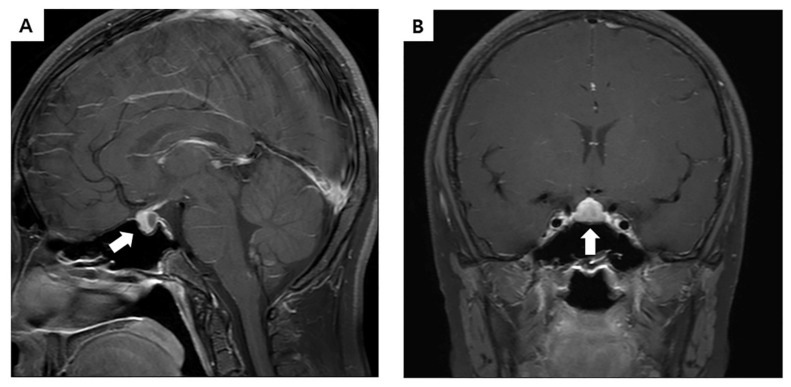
Sella magnetic resonance imaging (MRI) of the patient. T1 sagittal (A) and coronal (B) MRI showed a non-enhancing lesion in the posterior portion of pituitary gland measuring 8 mm in diameter with mild superior displacement of the optic chiasm (white arrow).
